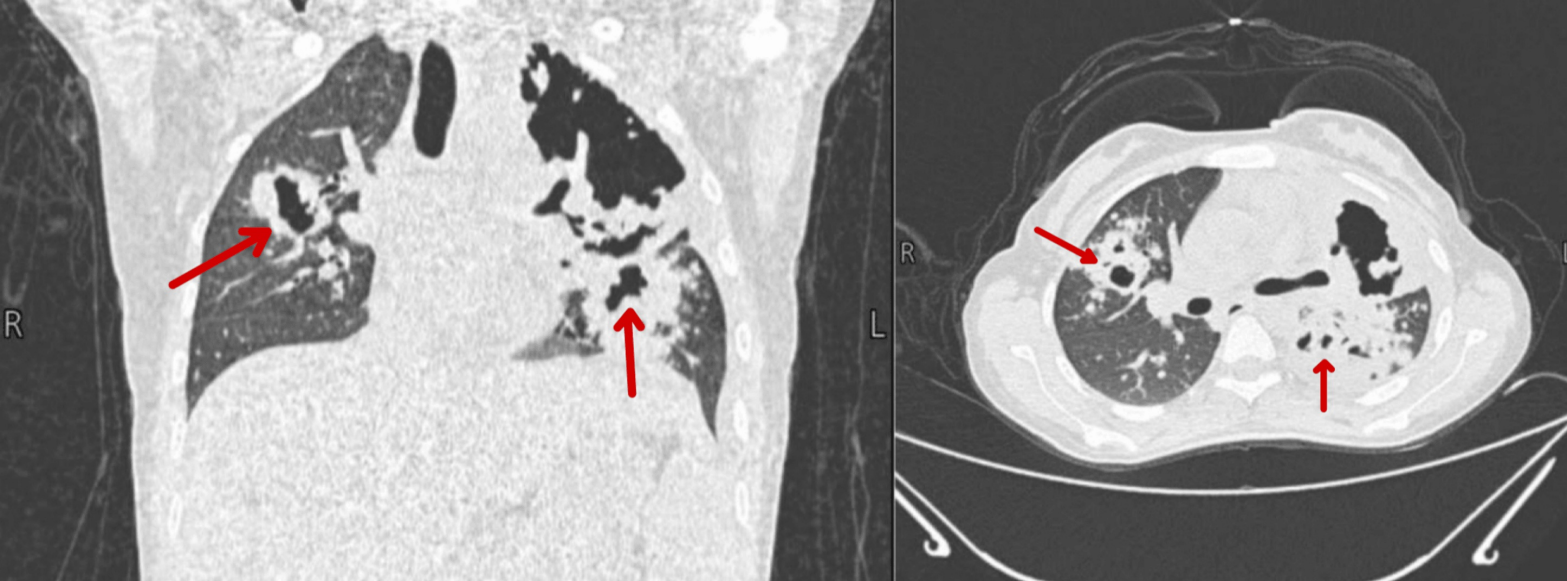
Chest CT scan revealed bilateral consolidation and a crazy paving pattern, accompanied by large cavities indicative of extensive parenchymal destruction, predominantly on the left side. Additionally, numerous small cavitating nodules were observed in all lobes, along with mild peripheral ground-glass opacity. There was also evidence of bronchial plugging at the lung bases.
